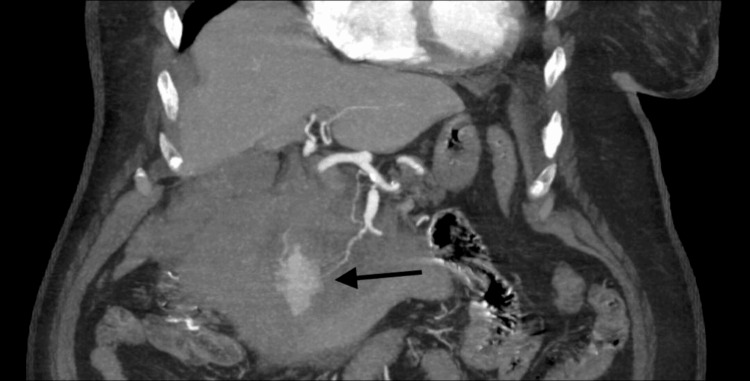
Computed tomography (CT) image demonstrating intra-abdominal hemorrhage with contrast extravasation
